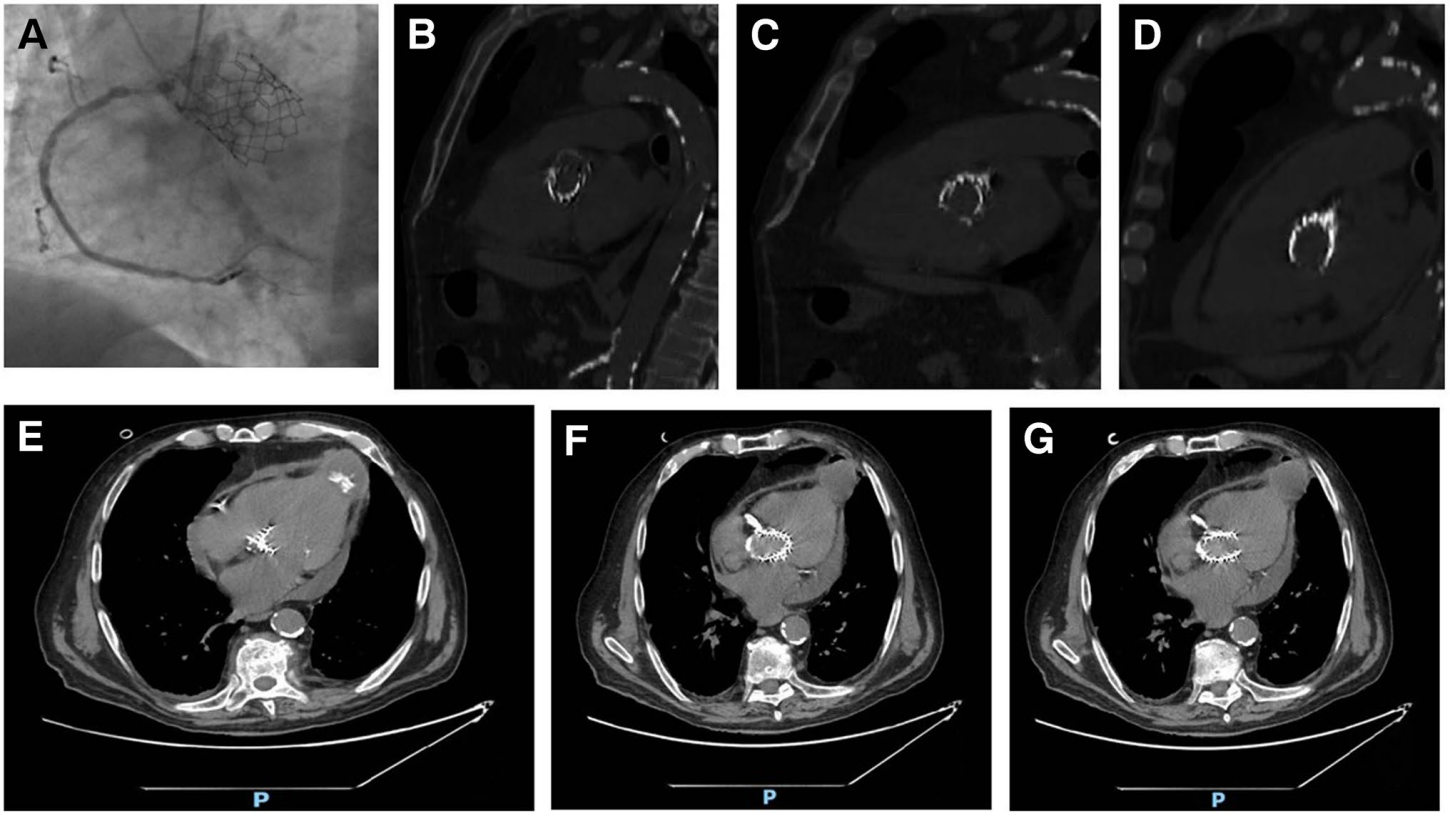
Coronary angiography and computed tomography images. Coronary angiography (A) shows the TAVI with the prosthesis correctly positioned. A computed tomography performed a few months after admission (B–D) for TAVI documented in the CT scans in the intrapericardial area, at the cardiac apex, the appearance of a 40 × 33 mm formation with partially calcified walls, the presence of fibrocalcified atheromatous aorta included in the acquisition volume, severe coronary calcifications, and a right coronary stent. The results of aortic valve replacement were confirmed. The CT scan performed during our admission (E–G) confirmed the presence of a “roundish image in the pericardial area at the apical level of the left ventricle to be referred to probable results of TAVI.” Based on these findings, we diagnosed a cardiac apex aneurysm as a result of TAVI intervention with trans-apical antegrade access
